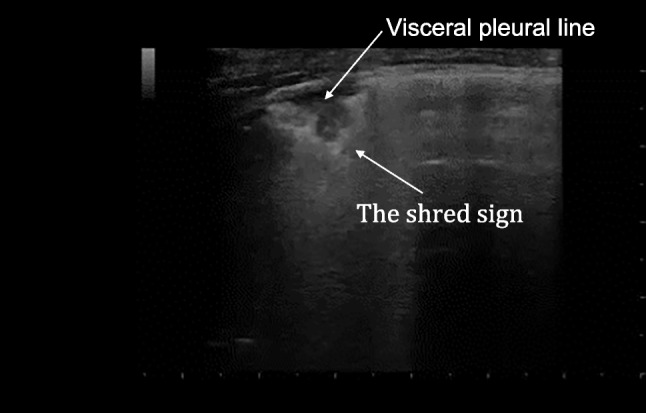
Lung ultrasound (LUS) finding: lung consolidation
